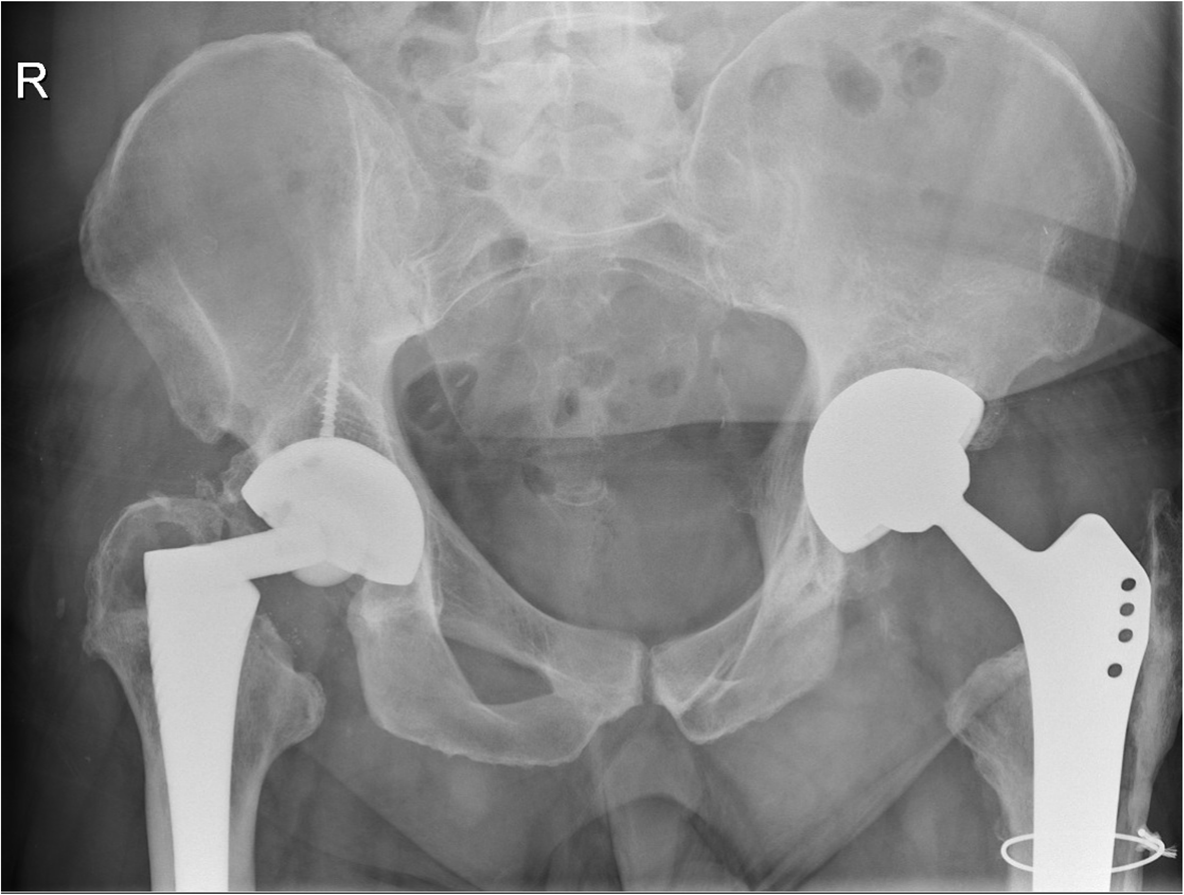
Pelvic X-ray with modular neck fracture shown on the right and situation 1 year after fourth revision of the left THA, respectively
